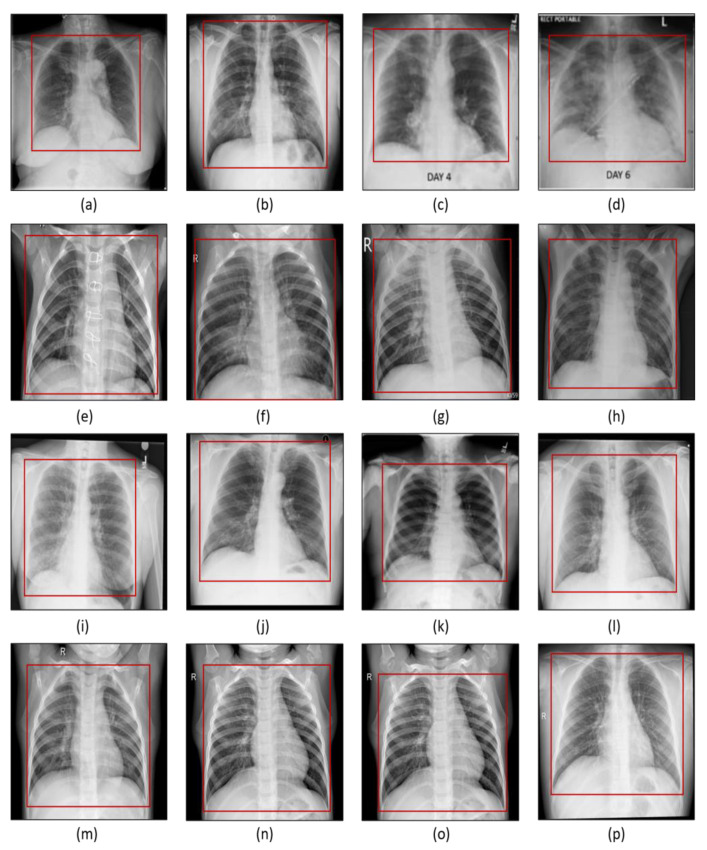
(a–d) COVID-19 chest X-ray, (e–h) pneumonia chest X-ray, (i–l) lung opacity chest X-ray, (m–p) normal chest X-ray. The red box signifies the region of interest cropped from the original image for analysis.
